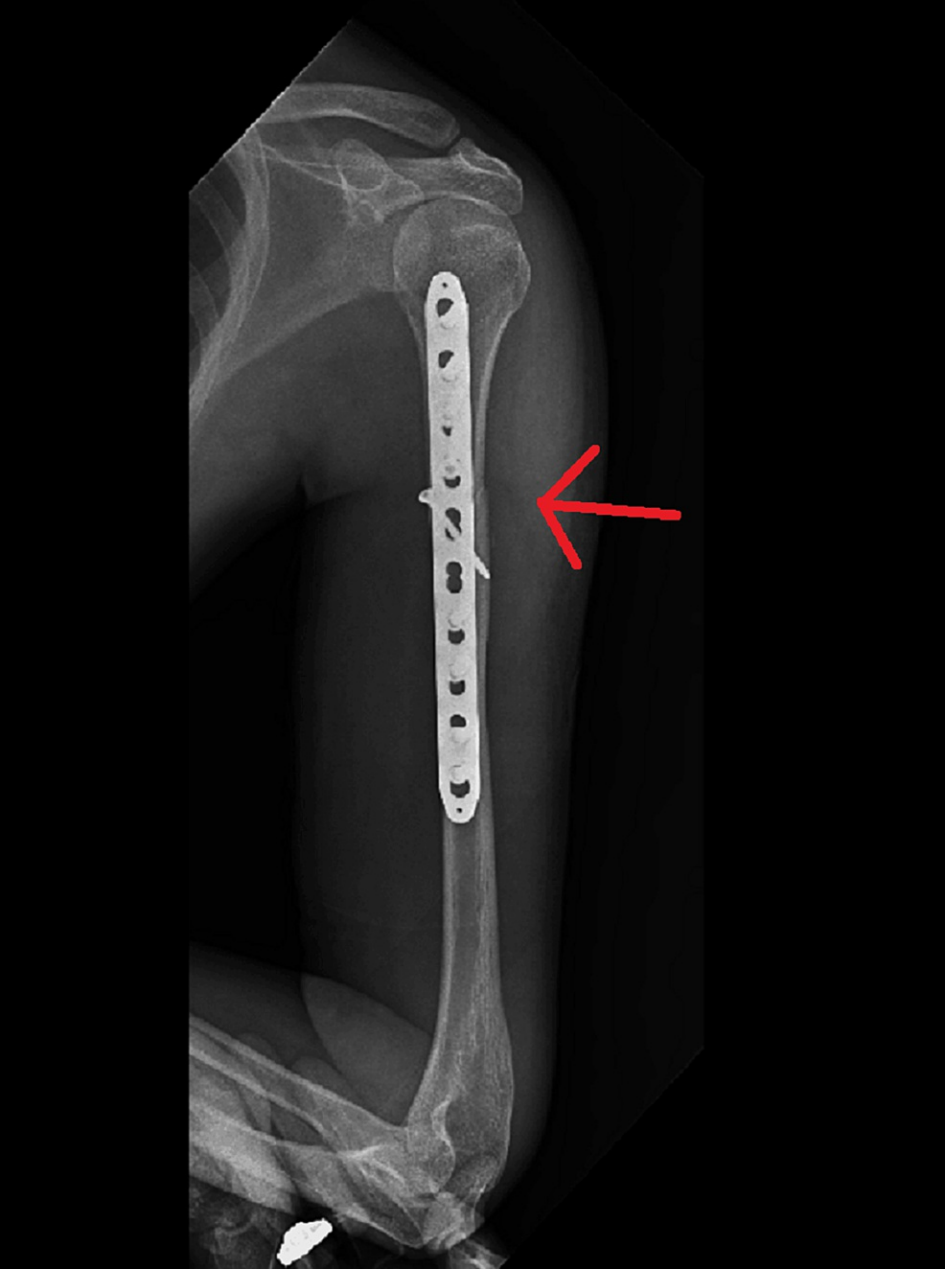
Lateral radiograph showing ORIF with plate osteosynthesis for proximal one-third humerus shaft fracture on left side ORIF - open reduction and internal fixation
